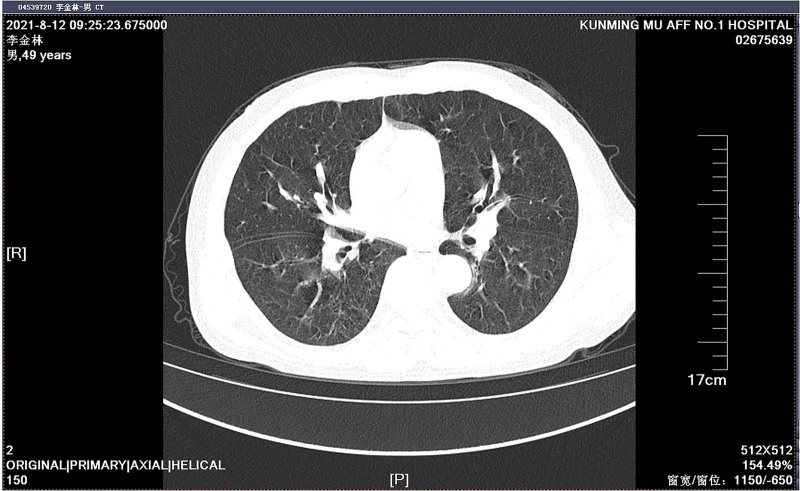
Chest CT on August 12, 2021 showed that multiple inflammatory consolidations in both lungs had been absorbed. CT = computed tomography.
